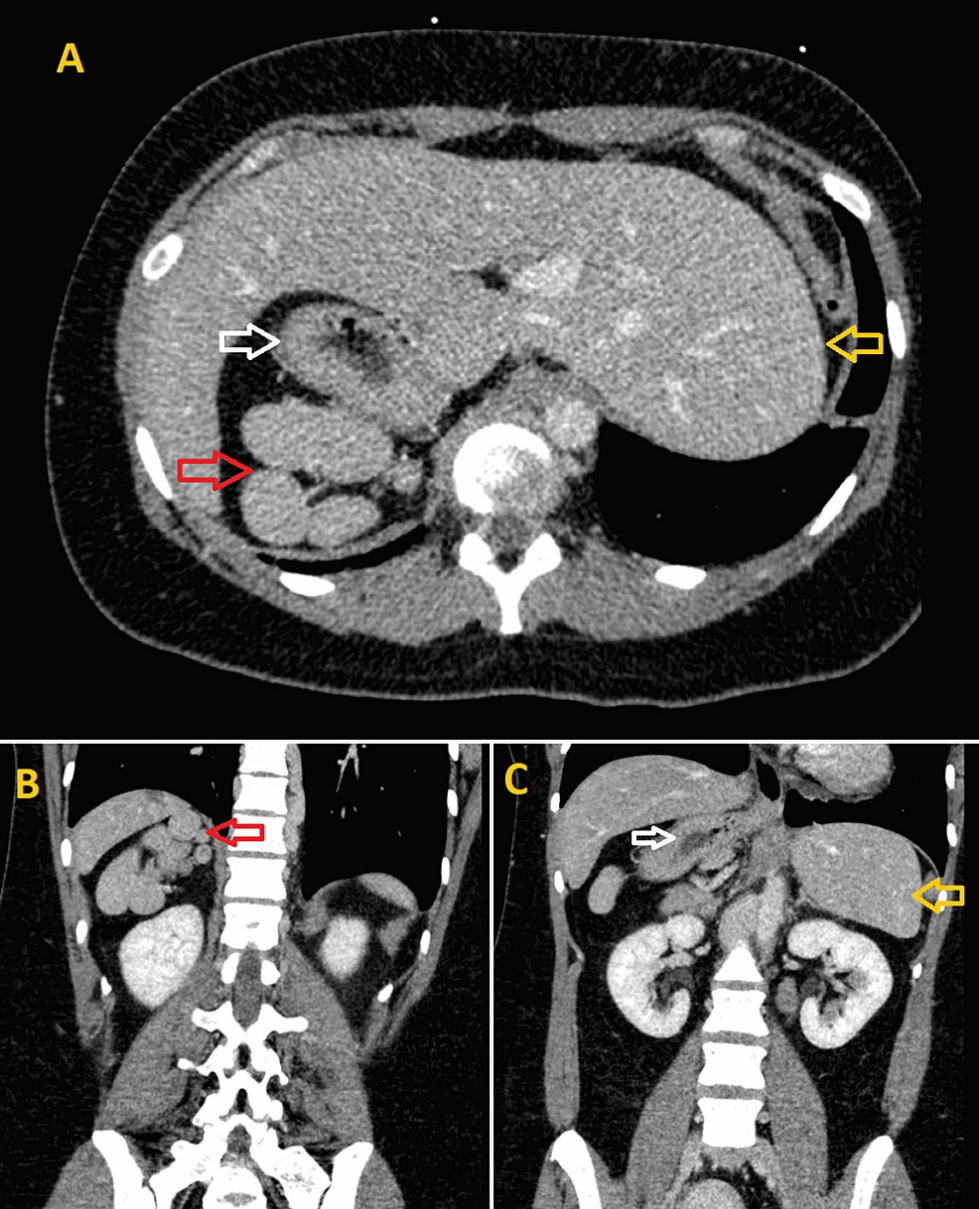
(A) Axial view and (B, C) coronal view of abdominal CT showing a right-sided stomach (white arrows), multiple splenules (polysplenia) without a patent spleen in the right upper quadrant (red arrows), and a prominent left hepatic lobe (yellow arrows), compatible with heterotaxy syndrome.
